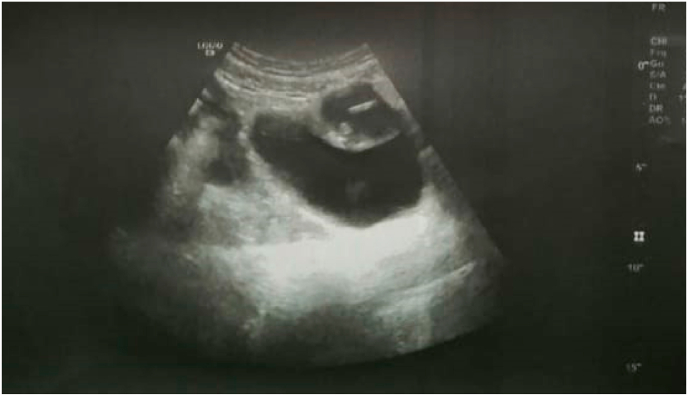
Suprapubic pelvic ultrasound: Appearance suggestive of a pre-vesical migration of an IUD with a collection around it.
